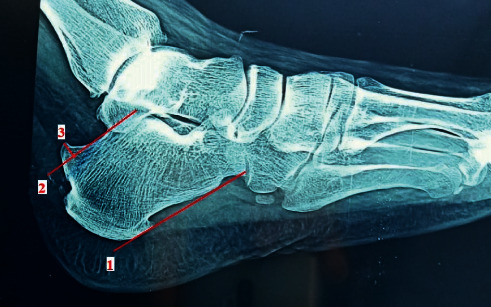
Length of bony exostosis is measured on lateral X-ray view of the calcaneus. Line 1 starts from the most inferior point of the calcaneocuboid along the plantar aspect of the calcaneus. Line 2 extends from the highest point of the subtalar and is parallel to line 1. Line 3 indicates the length of the bony hump above line 2.
